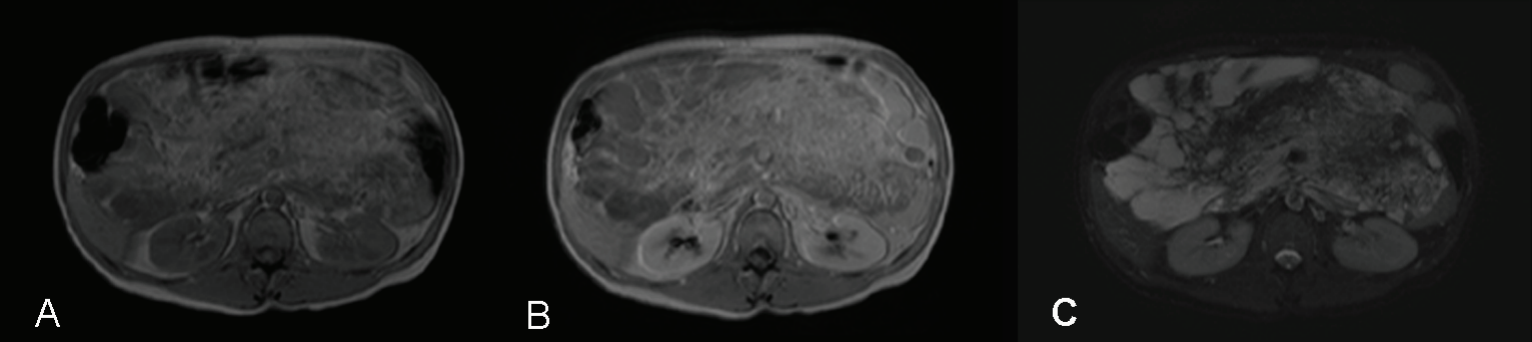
MR examination performed before (A) and after (B) contrast administration with T1- and T2-weighted images and suppression of fatty tissue (C) confirmed an abdominal mass with a dorsally located multicystoid area surrounded by fat.
